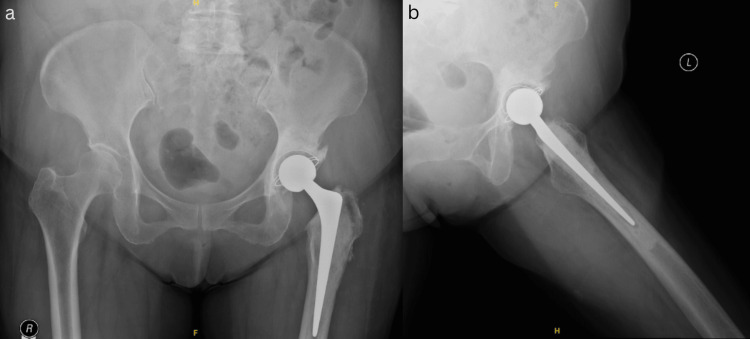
Post-operative X-rays of the pelvis in AP view (a) and left hip in lateral view (b)
